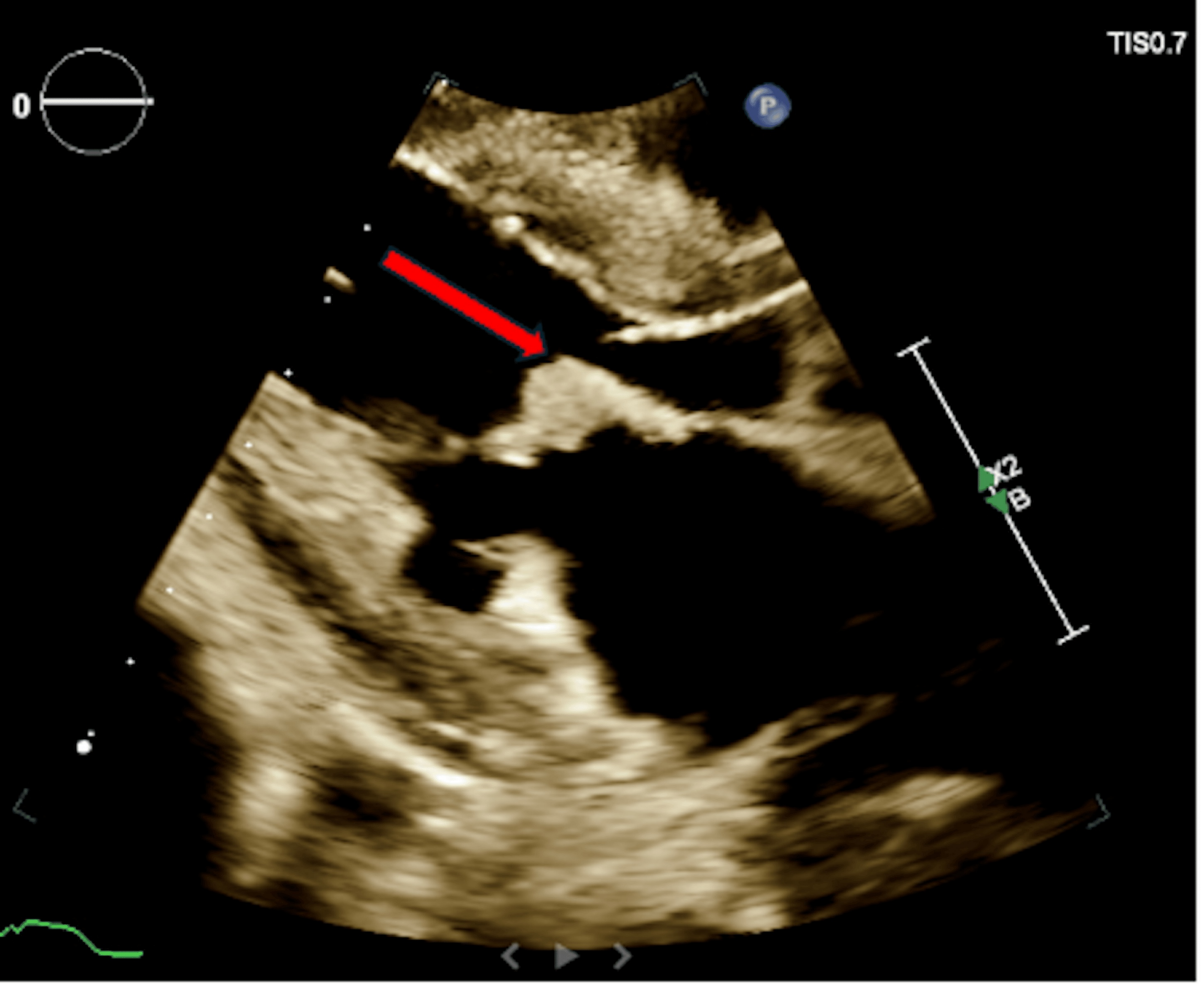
Parasternal long axis: thickening of the mitral leaflets with a mobile mass attached to the anterior leaflet of the mitral valve
